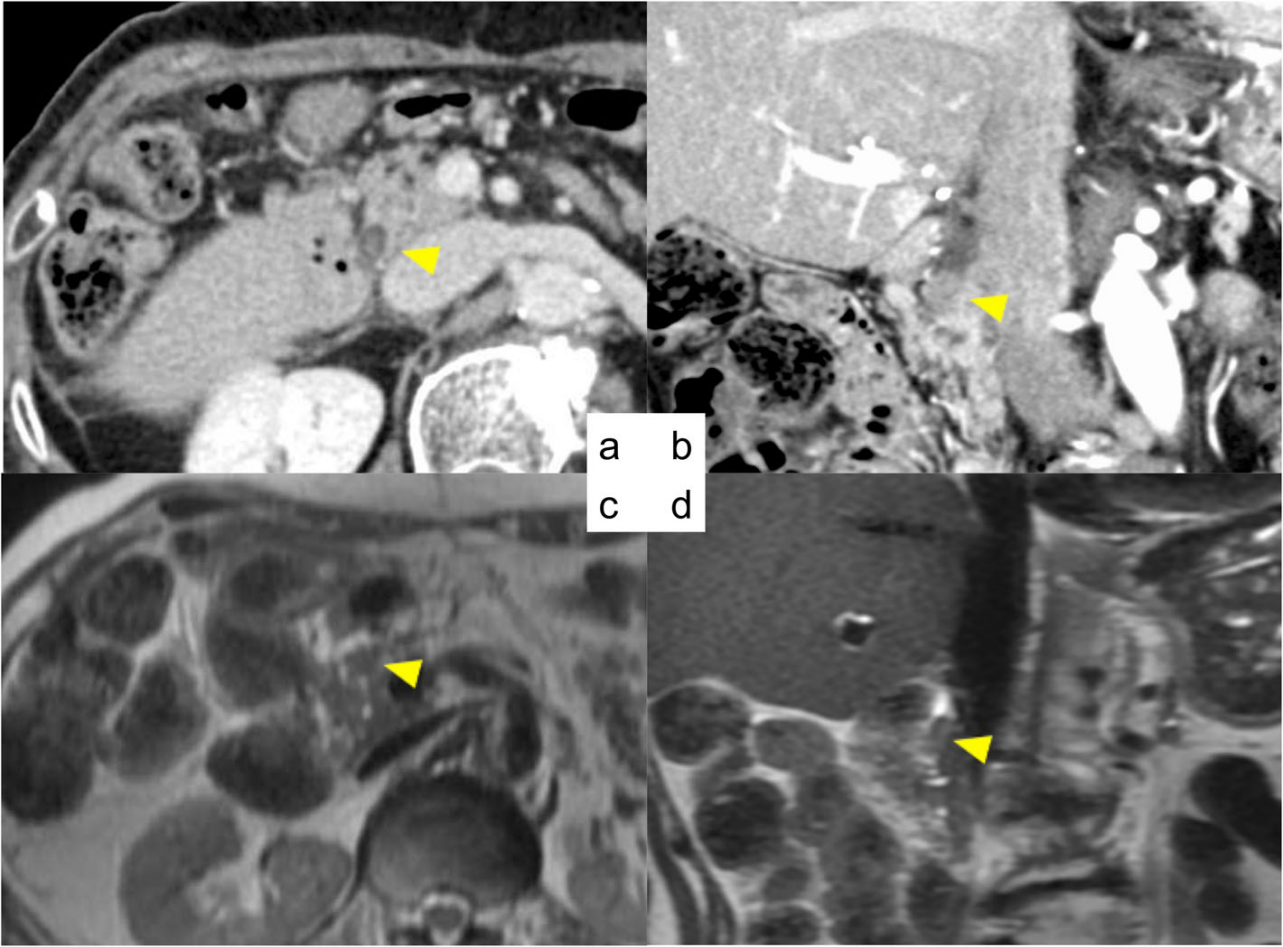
a, b Contrast enhanced CT scan revealed a 15 mm mass with enhancement in the early phase at the common bile duct. c, d MRCP revealed a 15 × 6 mm low intensity mass at the end of the common bile duct
